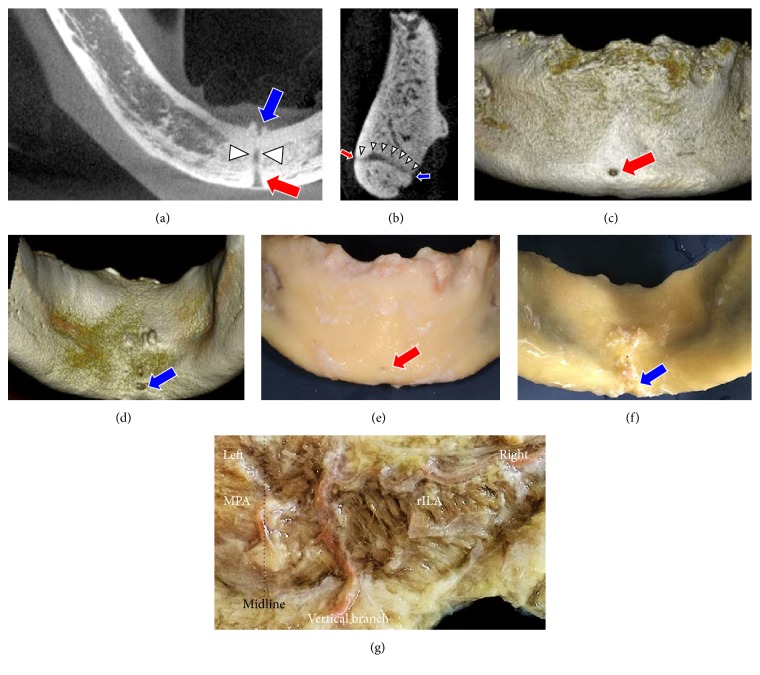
Axial (a) and sagittal (b) images of the mandible show two foramina located on the anterior (red arrow) and posterior (blue arrow) surfaces of the midline. A canal (white arrowheads), which has the continuity of those two foramina, was demonstrated. Three-dimensional computed tomography images of the mandible show anterior (c) and posterior (d) views of the mandible. After removing the overlying soft tissue, the mandible is seen in anterior (e) and posterior (f) views. The medial view of the dissected soft tissue after periosteal detachment shows the right ILA running horizontally and joining the vertical branch, which passes by the inferior border of the mandible (g). The median perforating artery (MPA) has no anastomosis with other branches. Red arrow, median labial foramen (MLaF); blue arrow, median lingual foramen (MLiF); rILA, right inferior labial artery.
